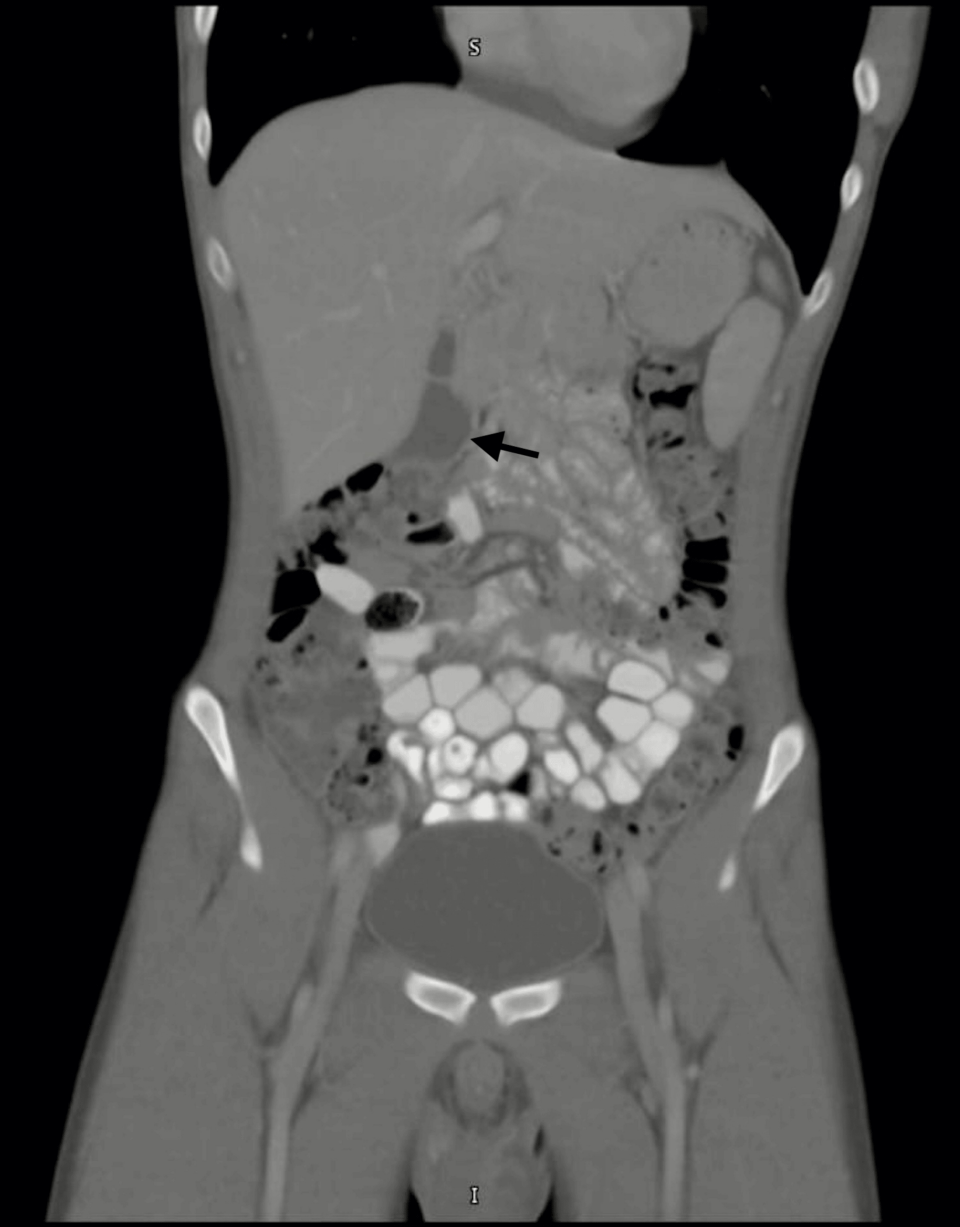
Contrast-enhanced CT abdomen demonstrating gallbladder wall enhancement with minimal hepatorenal free fluid. Contrast-enhanced computed tomography of the abdomen and pelvis showing mild reactive enhancement of the gallbladder wall and minimal free fluid in the hepatorenal fossa. No focal hepatic or splenic lesions were identified. These findings are supportive of inflammatory changes associated with acute dengue infection.
